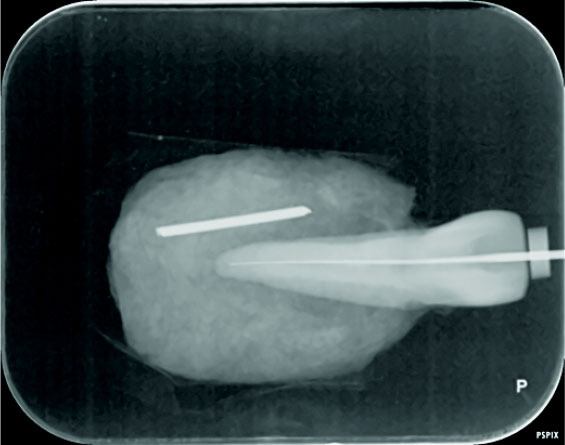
Digital radiograph with edge enhancement technique
